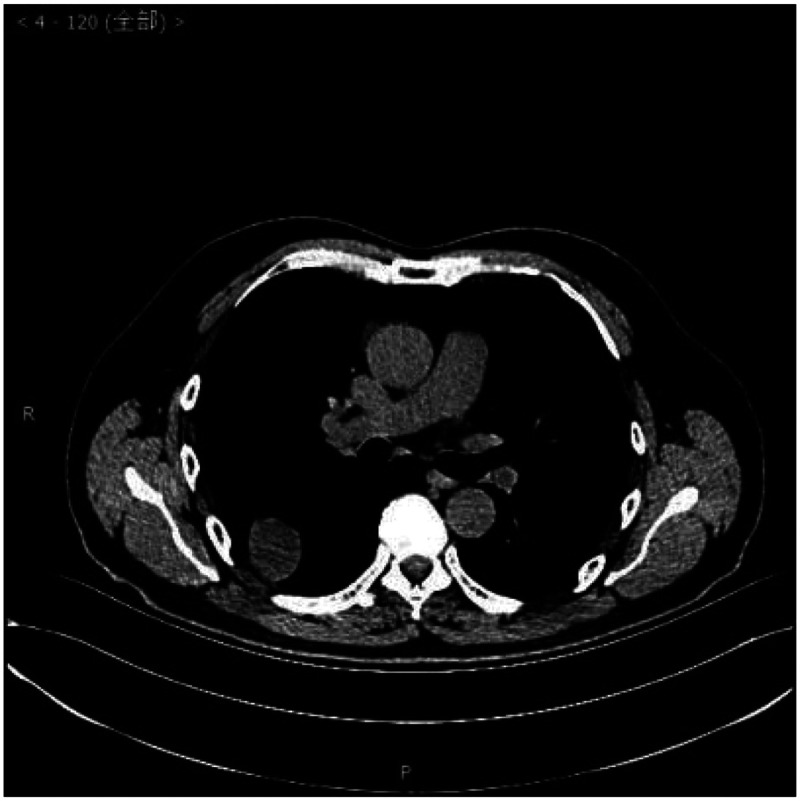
Chest computed tomography scan.
